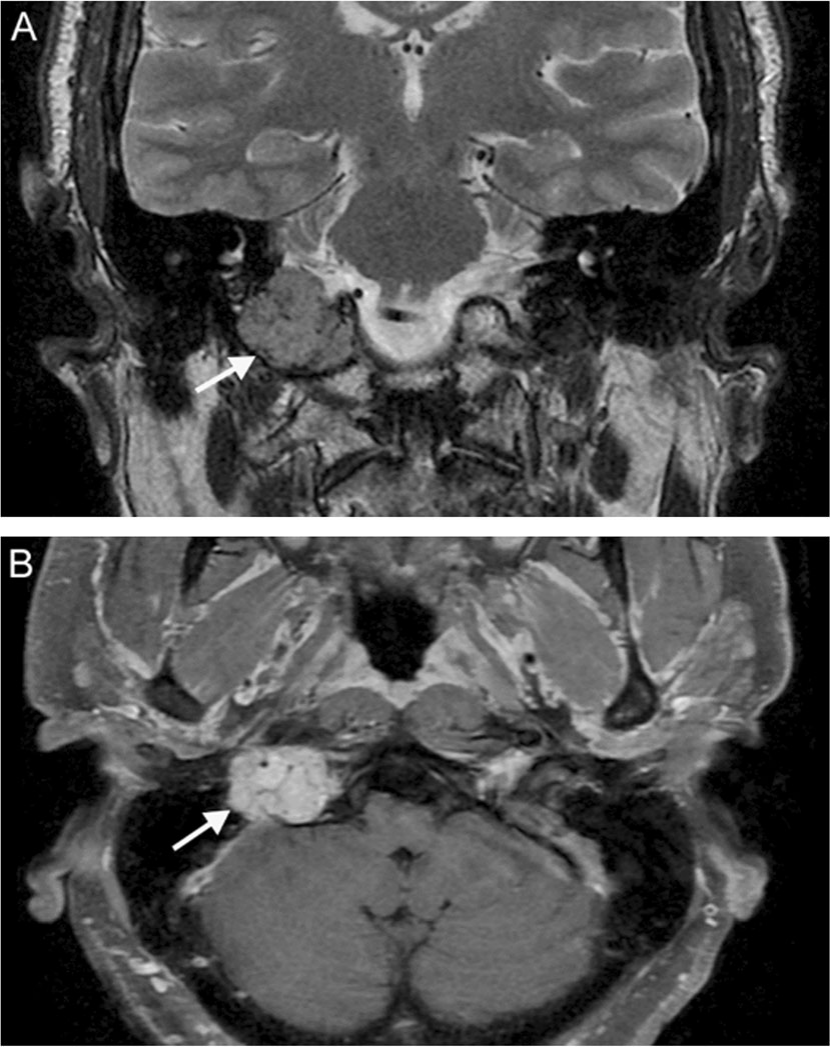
Jugular paraganglioma. A 55-year-old woman with relatively sudden onset hoarseness and dysphagia to liquids, who was found to have right vocal cord paralysis on laryngoscopy. Coronal T2 (a) and axial T1 post-contrast (b) MR images reveal a T2-isointense, avidly-enhancing lesion at the right jugular fossa (arrows) extending intracranially, with mild mass effect upon the right cerebellar hemisphere. Foci of low signal (“pepper”) represent flow voids, reflecting hypervascularity. Based on location and appearance, findings are consistent with a jugular paraganglioma (glomus jugulare tumor)
